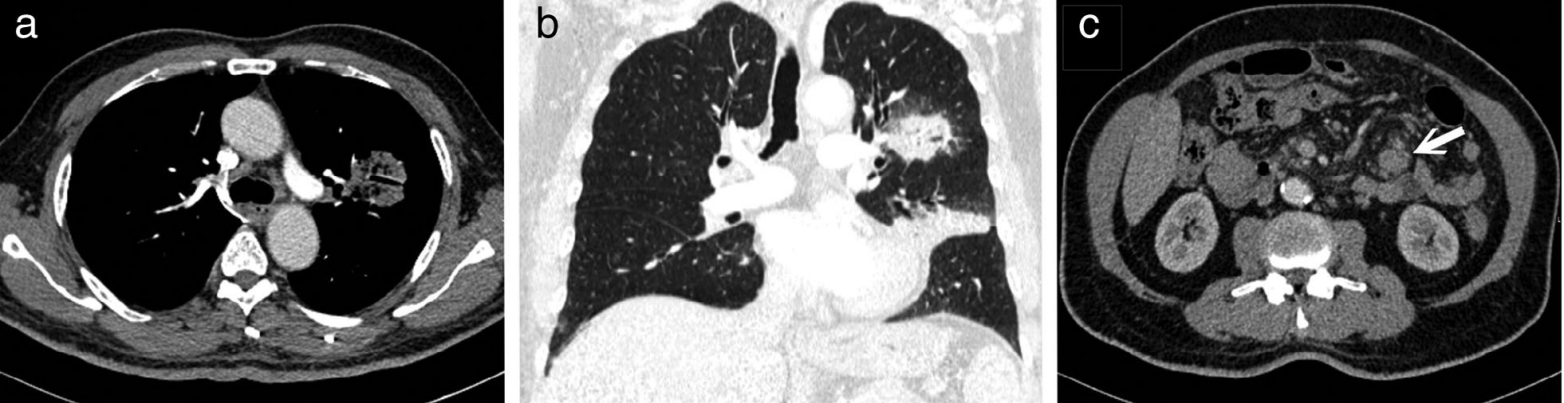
(a) Computed tomography (CT) scan of the chest demonstrated a 3.9 cm consolidative mass with air bronchogram in the left suprahilar region. (b) Lung window of a reformatted coronal plane image revealed ground‐glass opacities at the periphery of the left suprahilar mass and consolidation in the left inferior lingula. (c) The visible upper abdomen in the same CT scan showed a mildly enlarged mesenteric LN (arrow).
